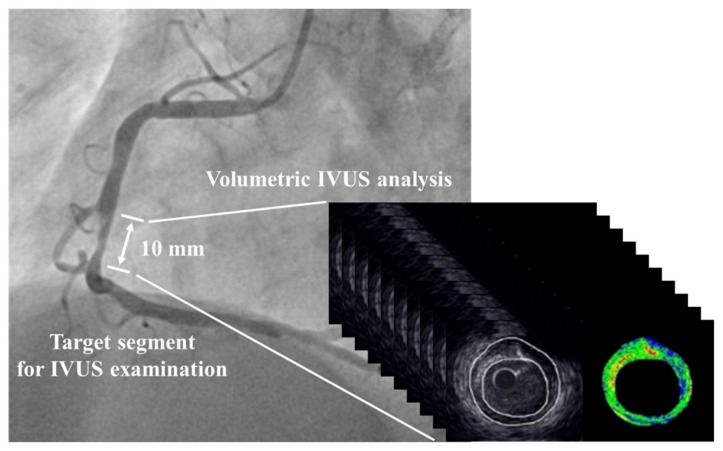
Representative Coronary Angiogram and IVUS Images. IVUS imaging was conducted just before PCI. A 10-mm target segment was defined based on the most-diseased cross section. Each cross-sectional image of IVUS and IB-IVUS spaced 1.0 mm apart. IB-IVUS = integrated backscatter intravascular ultrasound, IVUS = intravascular ultrasound, PCI = percutaneous coronary intervention.
